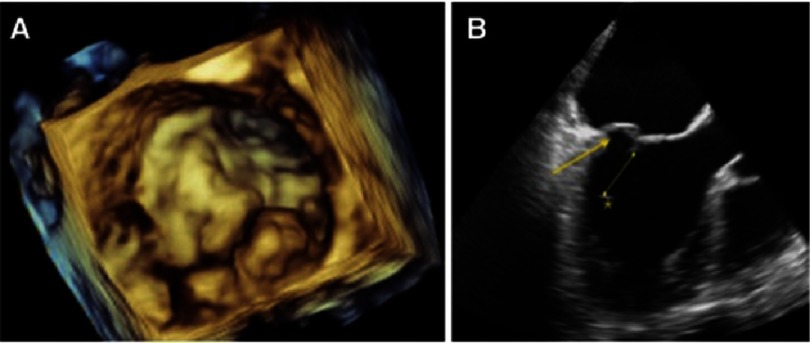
3D transesophageal echocardiography surgical view of P1-P2 segments of a mitral valve prolapse (A). 2D off line reconstruction from 3D full volume LV acquisition. Arrow: P2 prolapse, Line: Primary Chords elongation. Asterisk shows a papillary muscle tip displacement and high intensity echogenicity.
